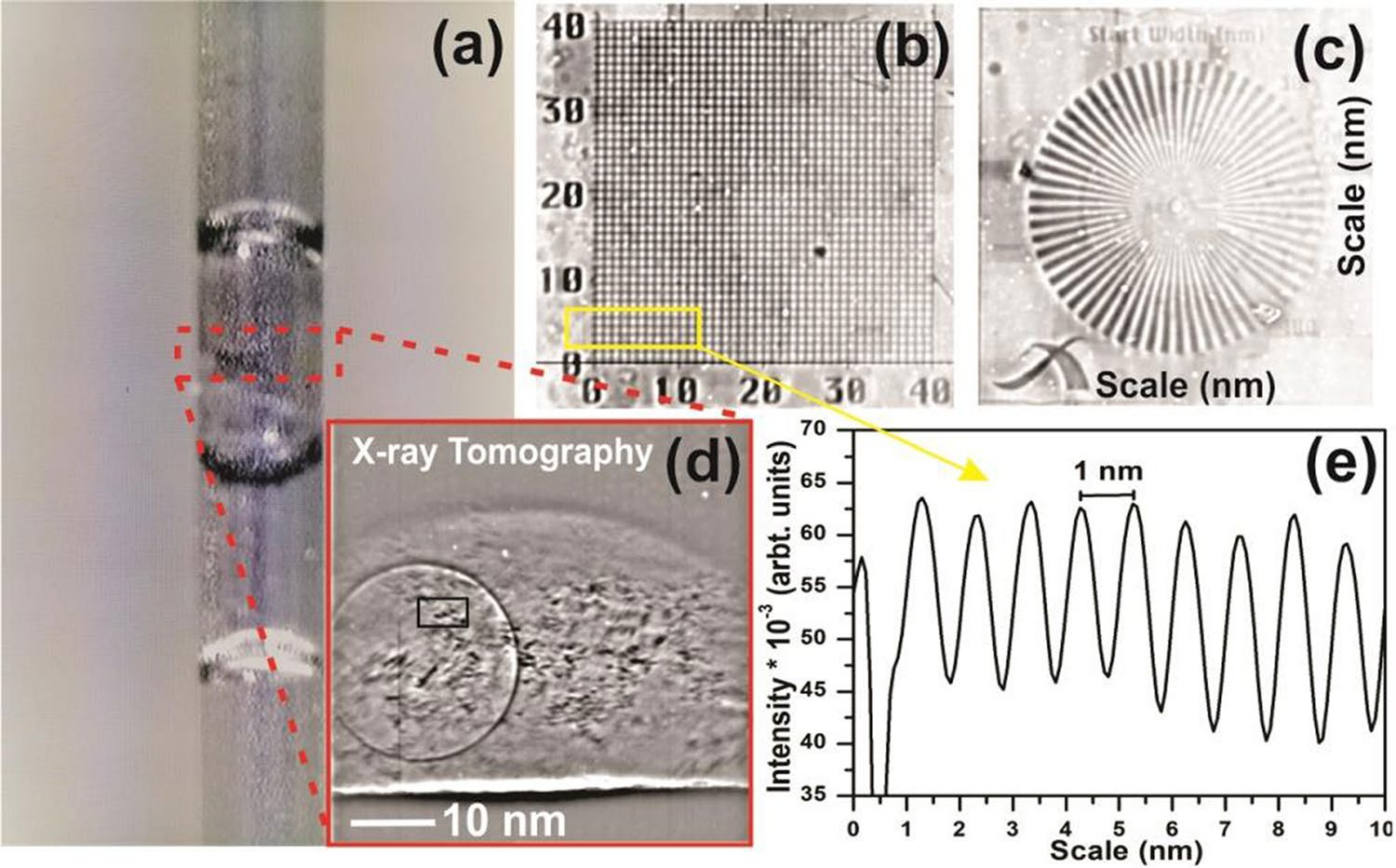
Nanotomography of plasmonic nanostructures: optical in-situ imaging of Kapton capillary filled with metal-salt precursor solution during the X-ray irradiation (a), X-ray imaging of scale pattern (b,c), in-situ X-ray imaging of Au-nanostructures (d), sectional line-scale pattern (e), respectively.
